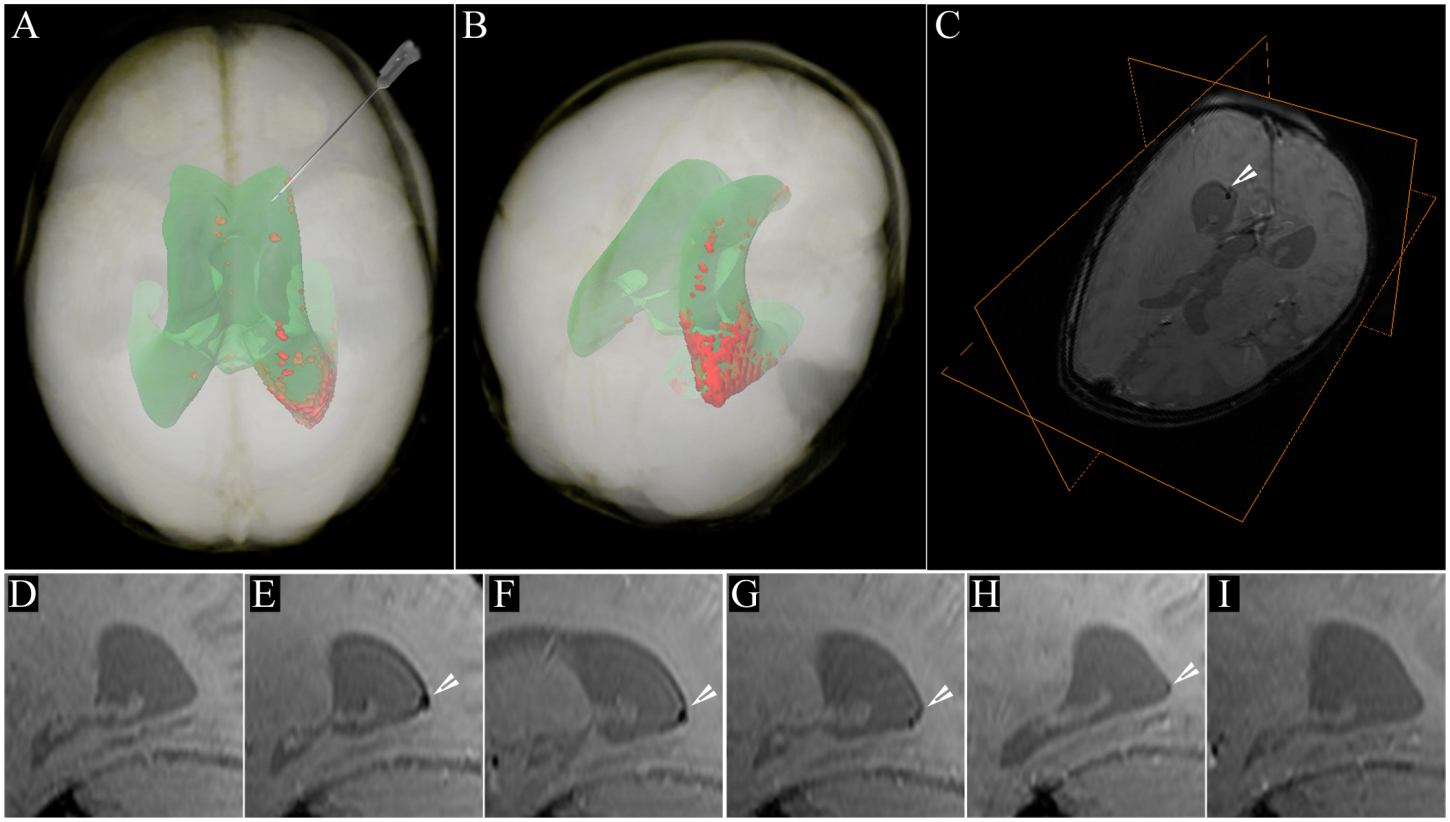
Imaging of SPIO-labeled autologous cord blood derived cells in a patient with global cerebral ischemia. A) Volume rendering of MRI data of the patient’s head obtained 24 hours post transplantation. Semi-automatic segmentation is based on pixel intensity, showing the projection of the ventricular system (*green*) and the distribution of the SPIO signal from the transplanted cells within the occipital horn of the right ventricle (*red*). Note the supine configuration of the head, corresponding to positioning during surgery. The route and trajectory of cell transplantation via the frontal horn is represented by the needle. B) Postero-superior view of the patient’s head, emphasizing the location of the hypointense SPIO signal from autologous cord blood derived cells transplanted within the occipital horn. C) T2*-weighted image with an orthogonal view centered on the cellular SPIO signal in the occipital horn (white arrowhead). D–I) Sagittal T2*-weighted MRI scans showing a longitudinal dispersion of SPIO signal within the occipital horn (white arrowheads); D) pre-transplantation, E) 24 hours post transplantation (PT), F) 7 days PT, G) 2 months PT, H) 4 months PT, and I) 33 months PT.
